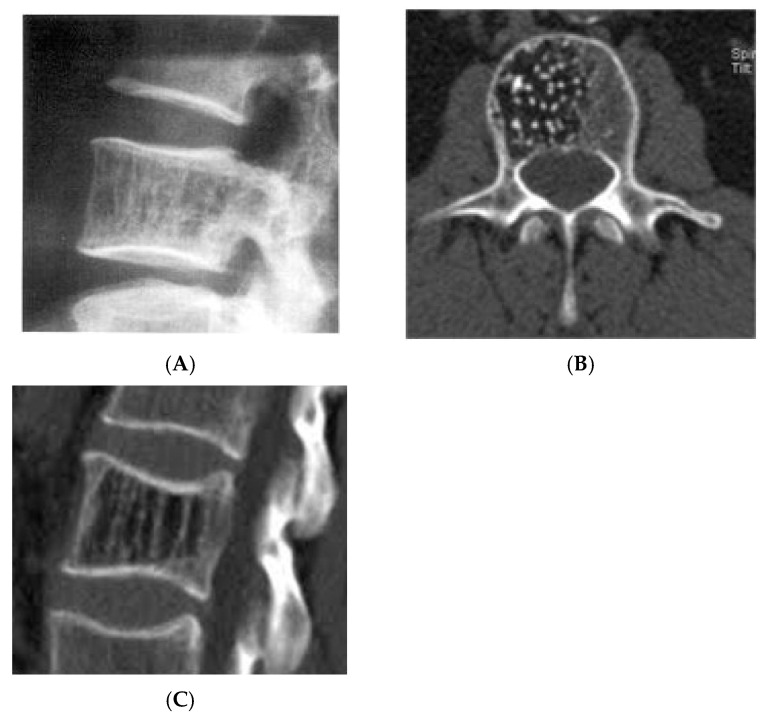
Vertebral haemangioma. (A) Plain radiograph lateral view demonstrating “corduroy” pattern. (B) Axial CT demonstrating “polka-dot” pattern. (C) Sagittal CT demonstrating “corduroy” pattern.
